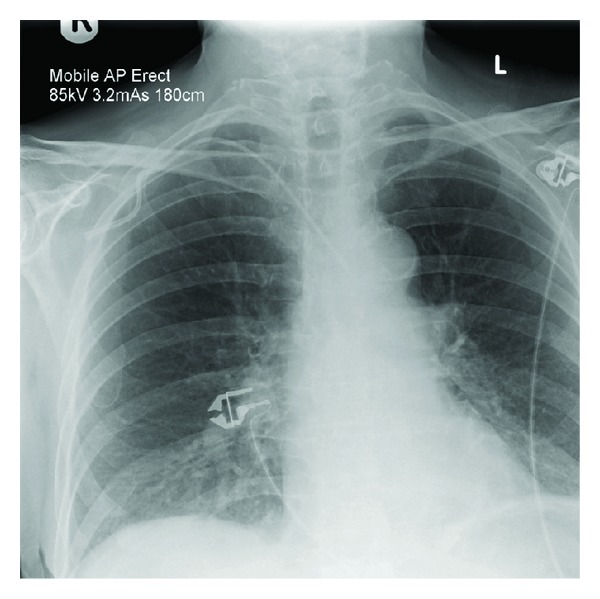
Chest radiograph showing abnormal position of the right subclavian line.
